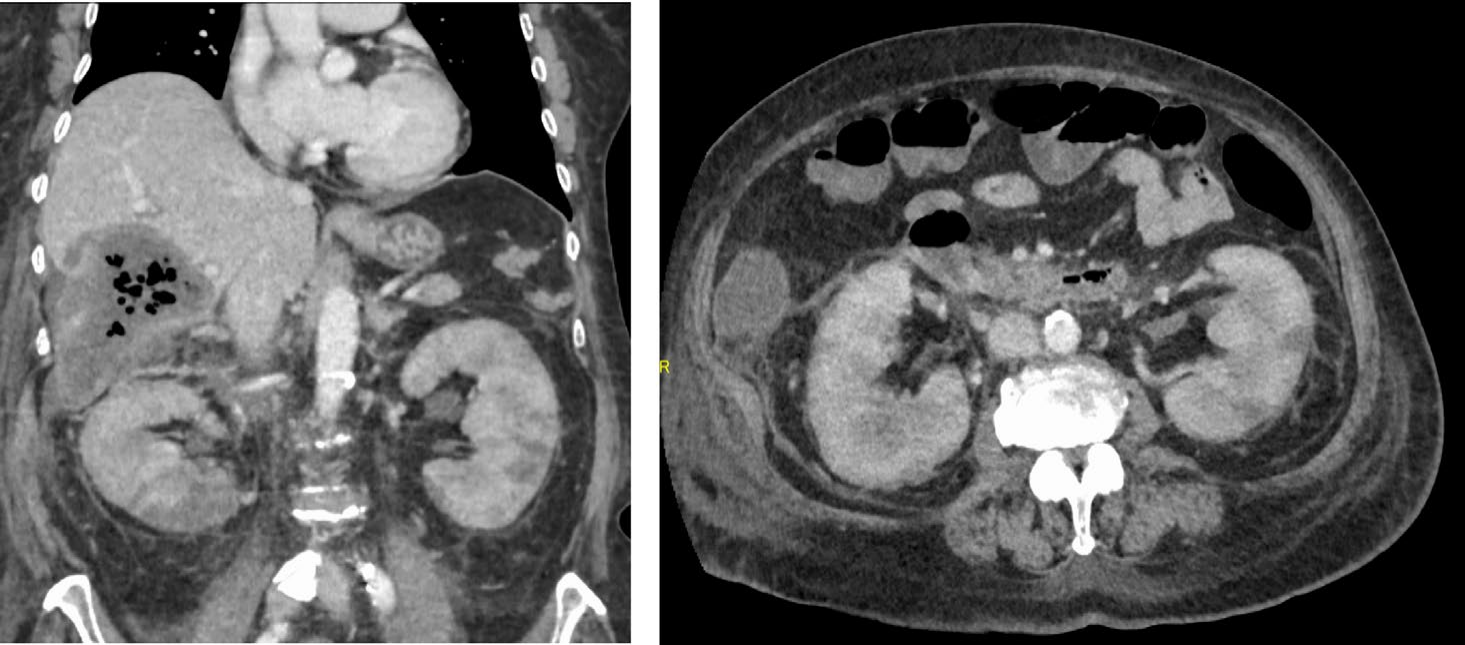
Abdominal enhanced computed tomography. A contrast computed tomography scan demonstrated a giant liver cyst and bilateral renal enlargement with poor contrast area.
